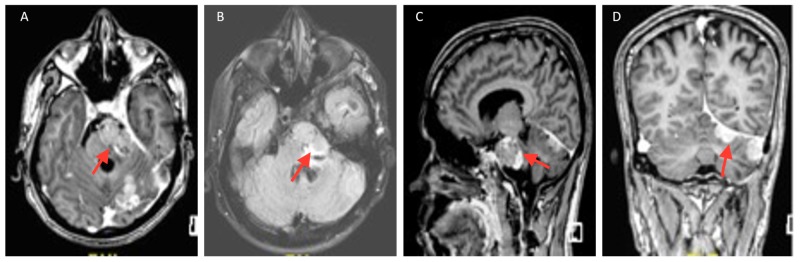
Magnetic resonance images (A) T1 Gado, (B) T2 Flair, (C) T1-weighted sagittal, and (D) T1-weighted coronal - multiple leptomeningeal and pachymeningeal T1 hyperintense areas were seen in both lobes of cerebral hemispheres.
